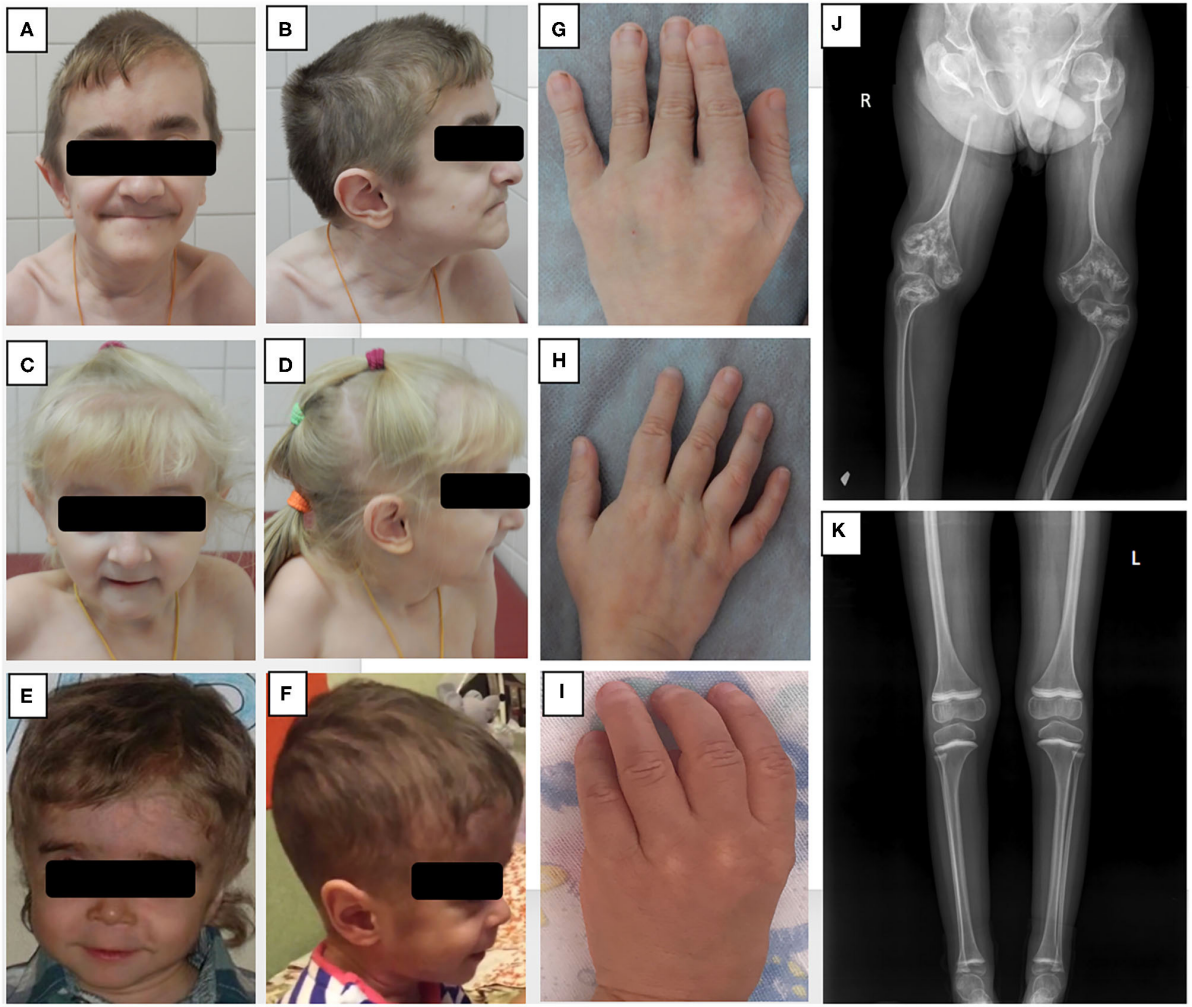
Phenotypic features and bone radiographs of three patients with *NBAS* mutations. (A–F) Frontal and side views of facial appearance of Patients 1, 2, and 3 at ages 13, 6, and 5, respectively. Note short neck and similar facial dysmorphism, including senile face, bilateral exophthalmos, low-set ears, narrow forehead, pointed chin, and small mouth with thin lips. (G–I) Brachydactyly and small hands of Patients 1, 2, and 3. (J, K) (J) X-ray of lower extremities of Patient 1 showing severe osteoporosis, gracile bones, signs of multiple fractures with non-union, and “popcorn” epiphyses; (K) X-ray of Patient 2 demonstrating mild osteoporosis with no axial deformities.
